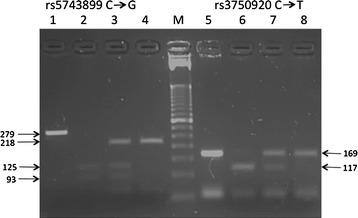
The rs5743899 and, rs3750920 polymorphisms of the *TOLLIP* gene as detected by restriction enzymes *Hha*I and, *Msp*I respectively. The gel depicts the PCR product (*lane 1*), restriction patterns related to homozygozity GG (*lane 2*), heterozygozity GA (*lane 3*) and, homozygosity AA (*lane 4*) for the rs5743899 and, similarly for the rs3750920, PCR product (*lane 5*), homozygote CC (*lane 6*), heterozygote CT (*lane 7*) and, homozygote TT (*lane 6*). *Lane M*, DNA molecular-weight marker ladder 50 base pairs
